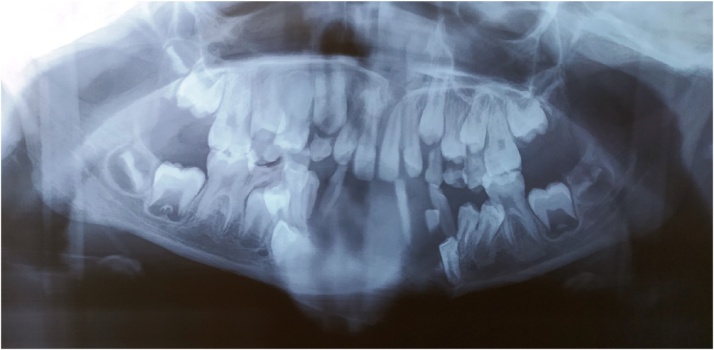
The panoramic dental x-rays showing central opacity with tooth displacement.
